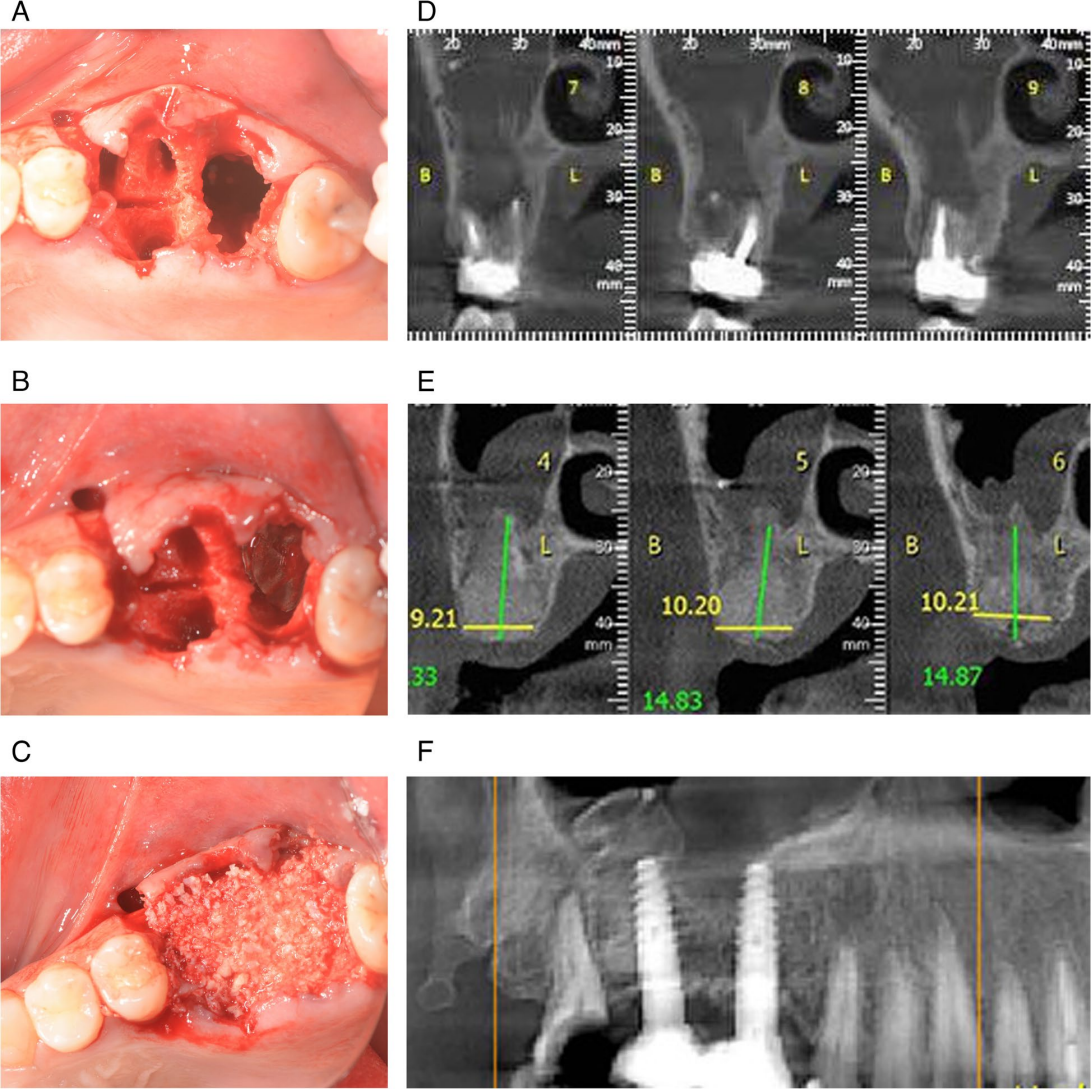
A After serial extraction of teeth 16 and 17, oroantral communication at the site of the maxillary second molar was clinically noted and diagnosed. B The magnesium membrane (black arrow) was folded and placed in a way that the communication was closed. C Allograft and xenograft granules were used for augmentation and a second magnesium membrane was placed over the augmentation. D Coronal preoperative CBCT shows that the roots of maxillary second molar were placed directly in the right maxillary sinus. E CBCT four months after graft placement shows that the graft has integrated with the bone and that there was sufficient buccal and palatal bone to place two implants. F Panoramic image 8 months post-operatively shows two integrated implants with no signs of peri-implantitis
